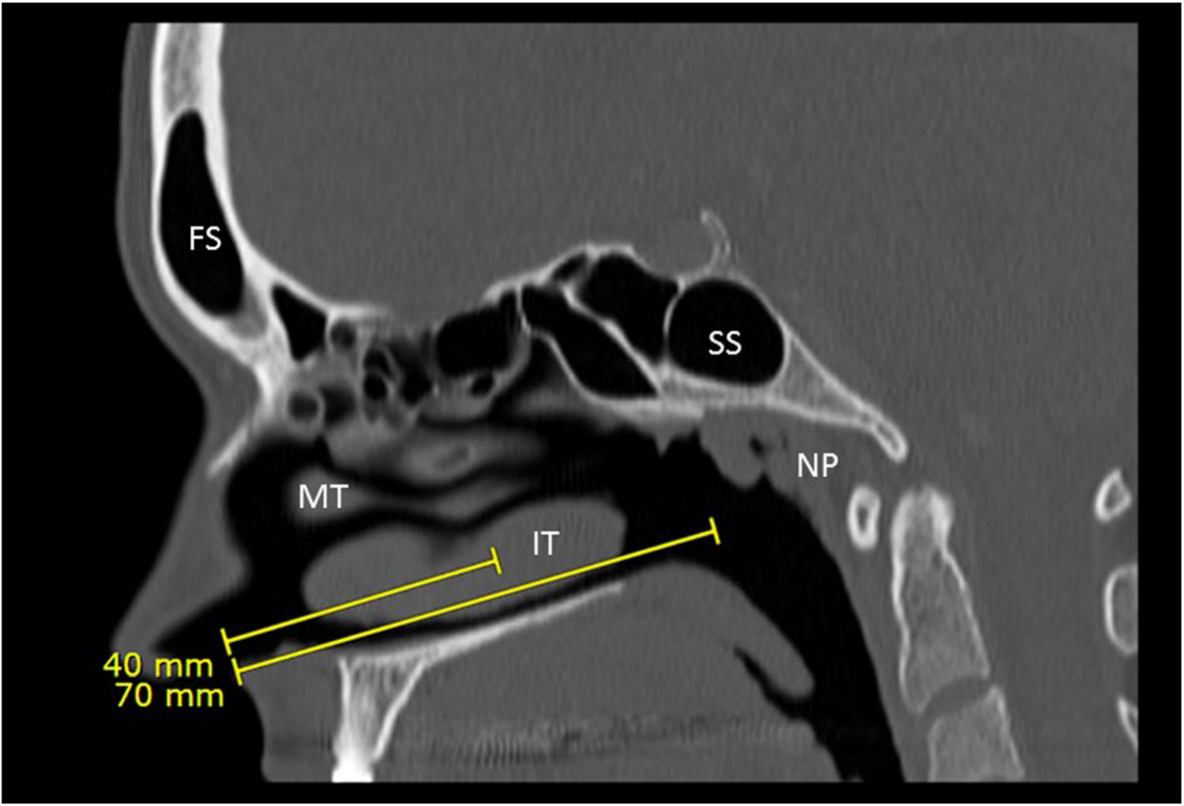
Sagittal CT view of nose and nasopharynx (50-year-old Caucasian female). Areas reached by recommended measured depth of insertion are demonstrated. FS - Frontal Sinus, MT - Middle Turbinate, IT - Inferior Turbinate, SS - Sphenoid Sinus, NP - Nasopharynx
